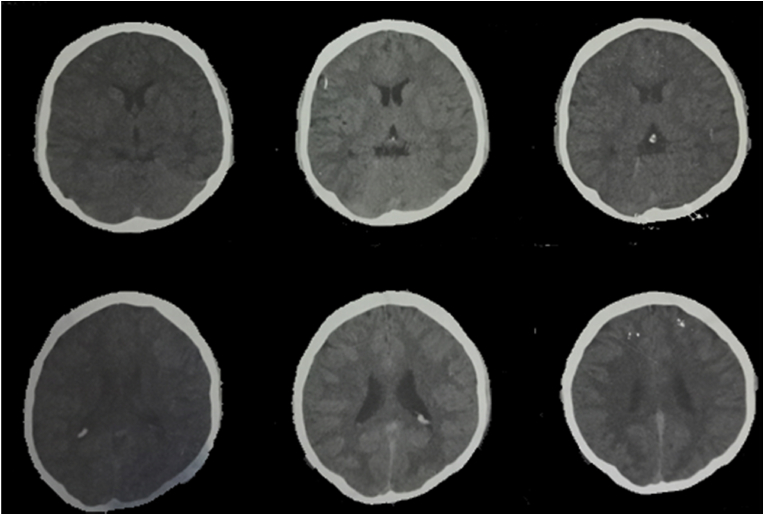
Non-enhanced CT Brain (axial) from the emergency department showing hypo-attenuation of the white matter and diffuse sulcal effacement.
